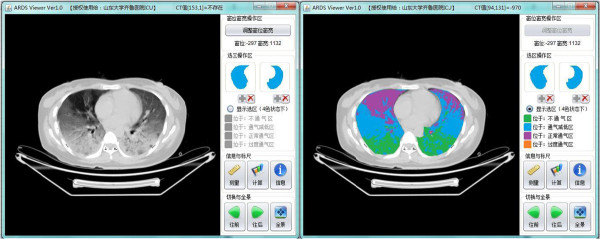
Computed tomographic scans obtained in a 32-yr-old patient with “patchy” acute respiratory distress syndrome caused by invasive aspergillosis. Contiguous 0.5-cm-thick computed tomographic sections were obtained from the apex to the diaphragm in positive end-expiratory pressure of 10 cmH_2_O. As shown by the ARDS viewer, the entire lung is composed of nonaerated (green), poorly aerated (blue), and normally aerated (purple) lung regions. The gas content (GV_CT_) is 506.86 ml, and the lung tissue volume (TV_CT_) is 1652.71 ml.
